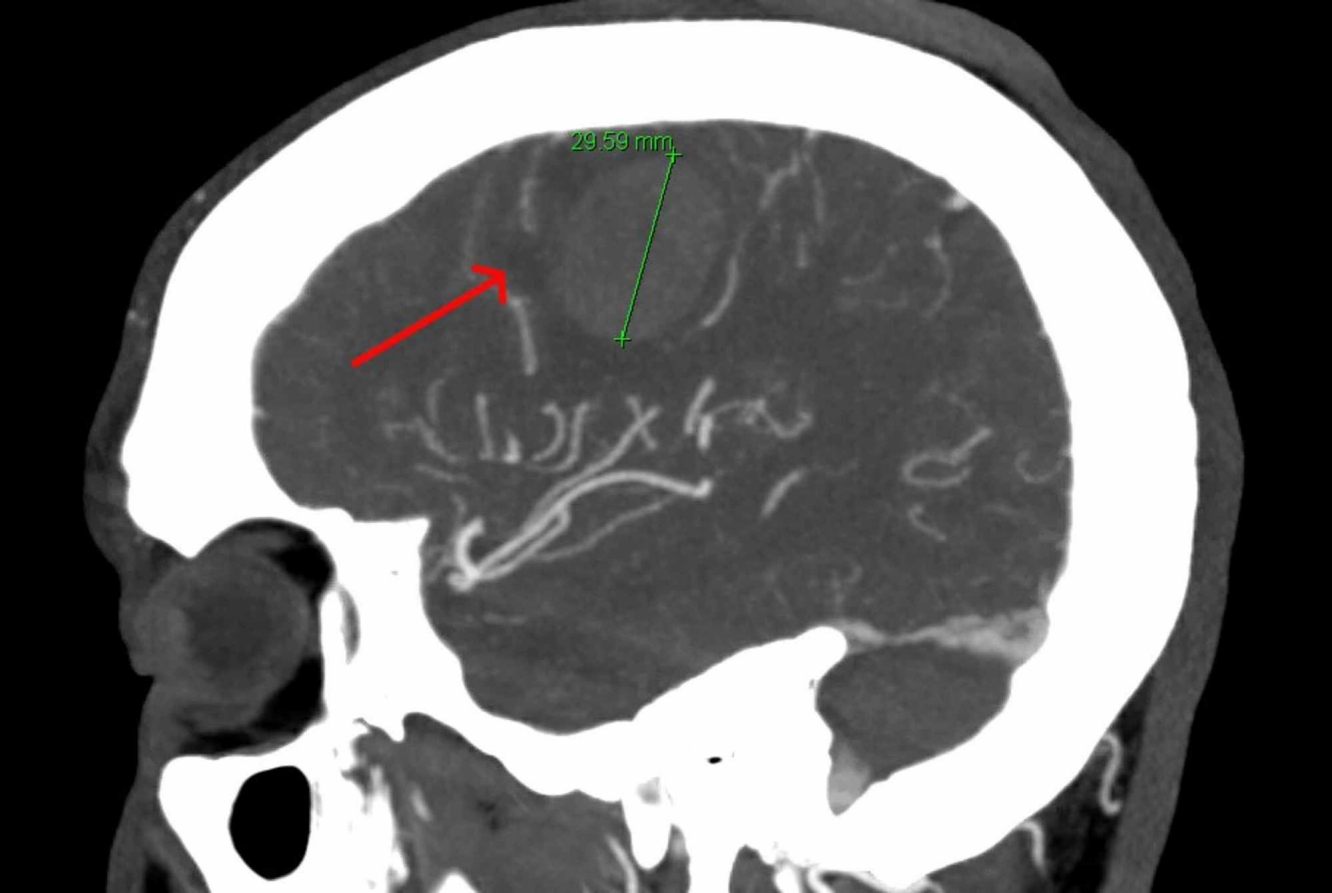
CT angiogram head and neck
